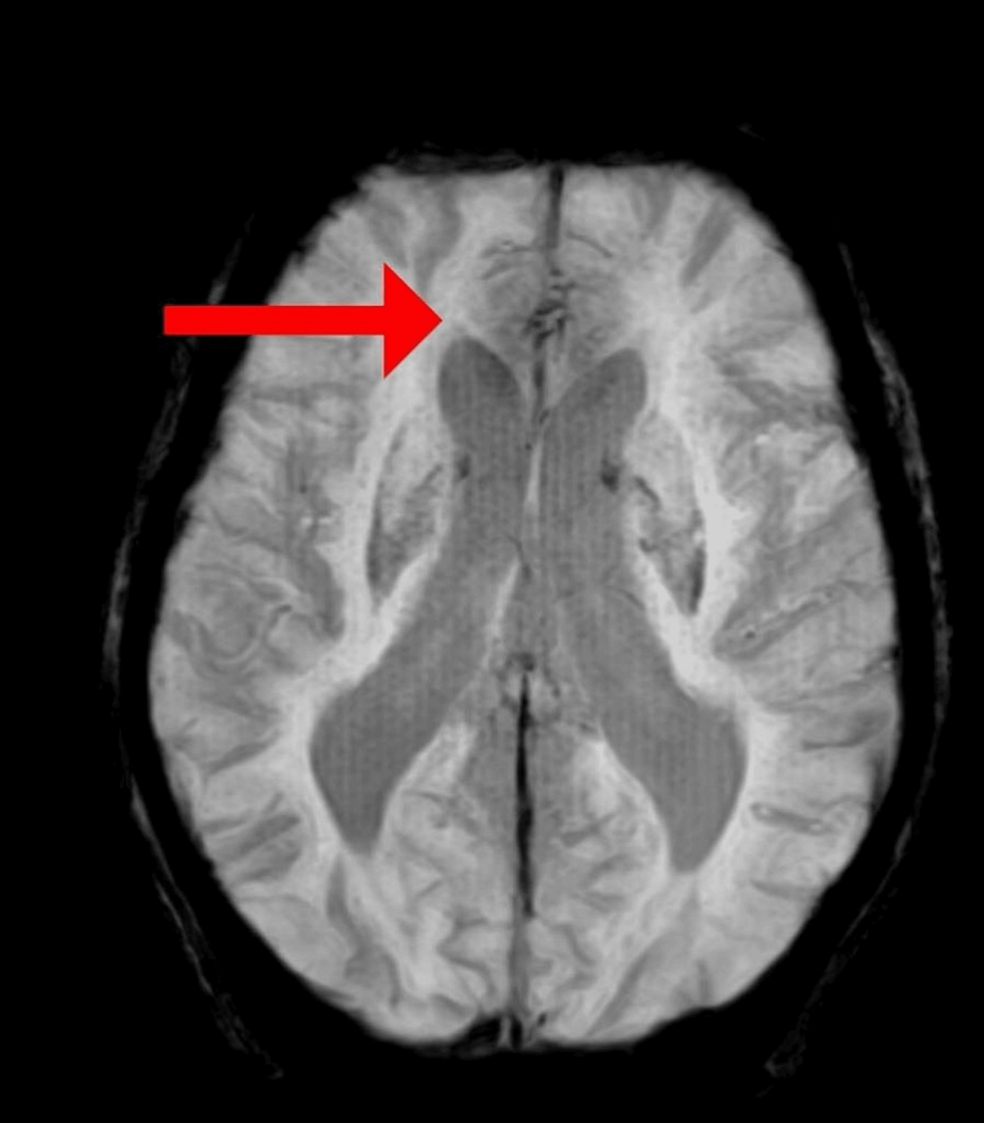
MRI showing bilateral periventricular deep white matter showing small vessel ischemic changes (red arrow)
